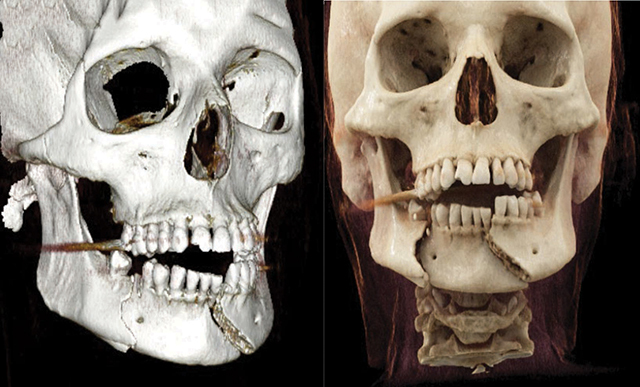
3D CT reconstruction showing a bifocal mandibular fracture with displacement.
